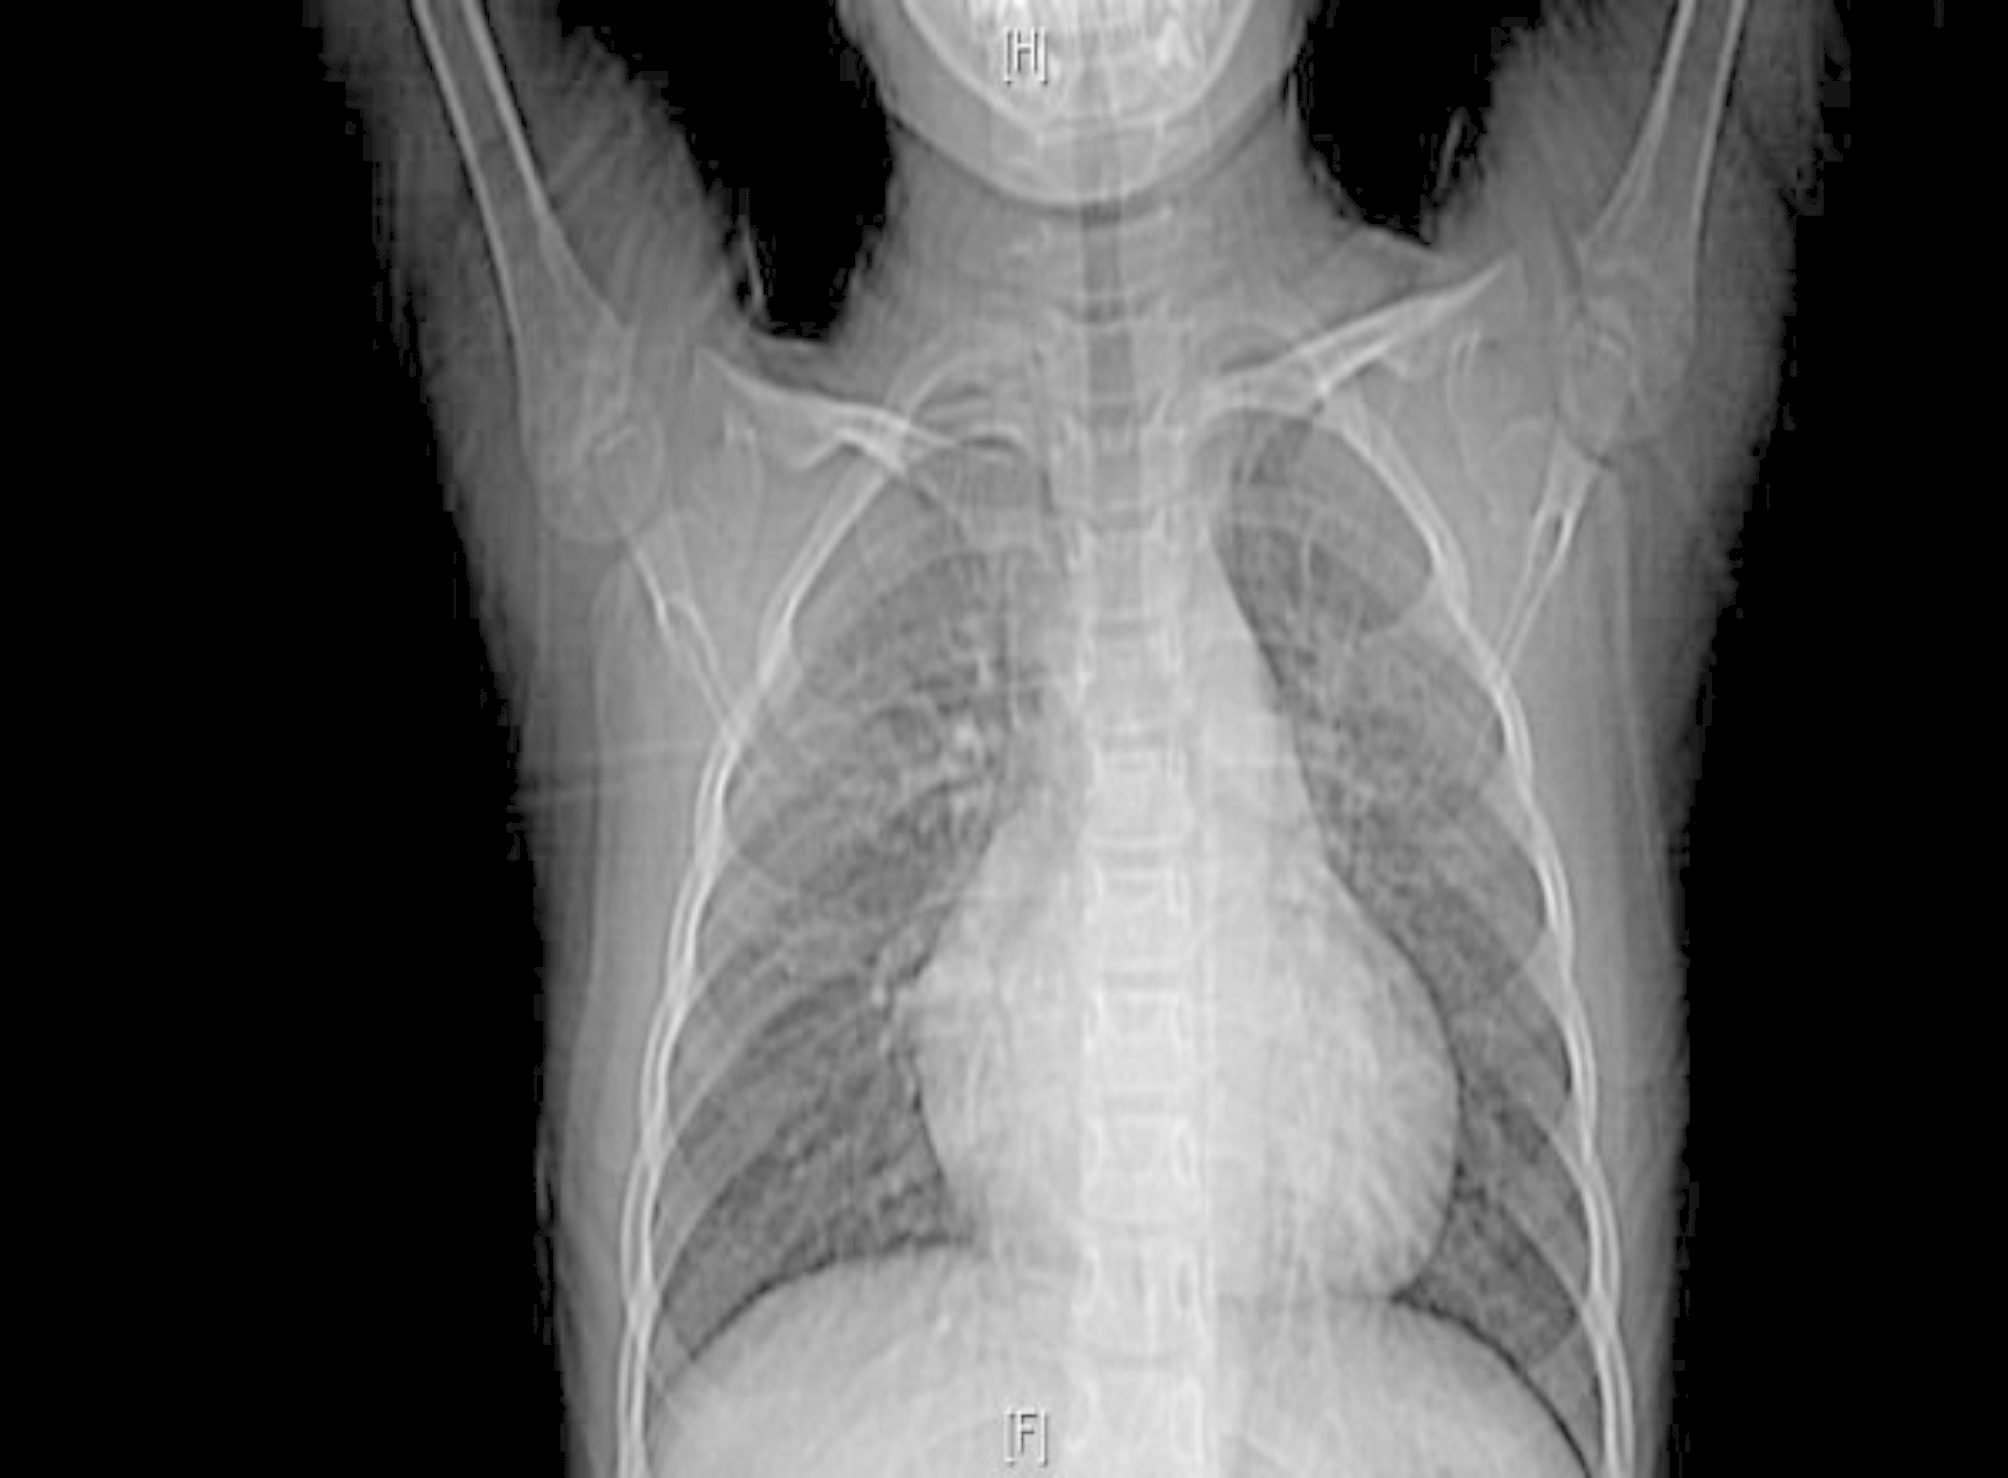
Preoperative chest X-ray revealed a large heart in the patient with cardiac myxoma
